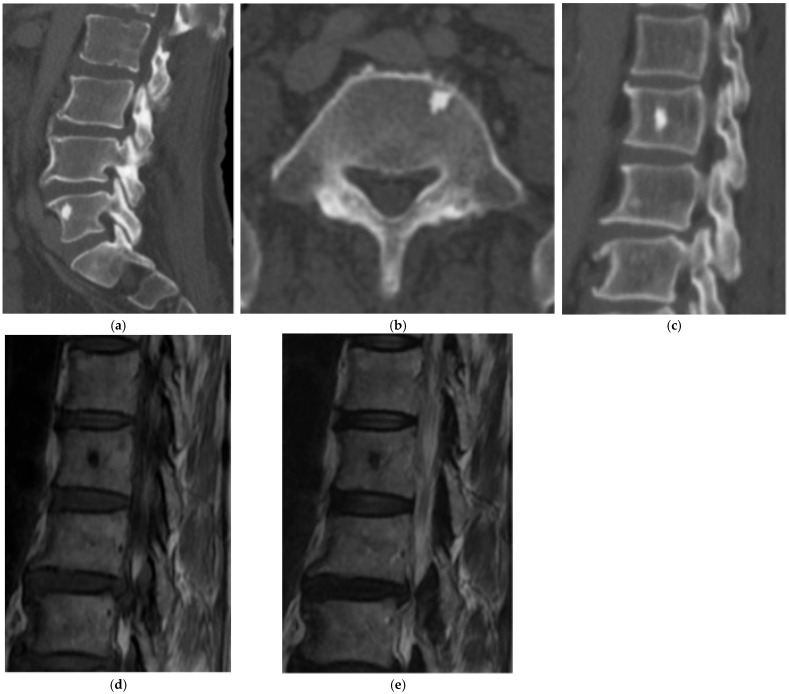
Enostoses/Bone Island. (a) Sagittal and (b) axial CT bone window demonstrate an enostosis (bone island) in the right anterolateral aspect of the L5 vertebral body. Radiating bony streaks are visualized along the periphery. (c) Sagittal CT bone window shows another enostosis in the lumbar spine centered within a vertebral body. (d) Sagittal MR T1 and (e) T2-weighted MR images show a focus of low signal intensity corresponding to the enostosis.
